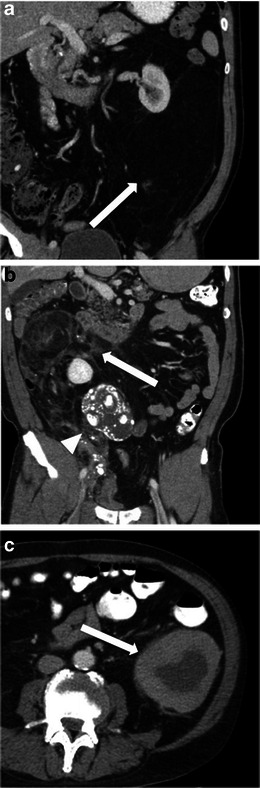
Imaging of liposarcoma a A 62-year-old woman with biopsy-proved well-differentiated liposarcoma. Note the large left retroperitoneal mass, which is of predominantly fatty attenuation (*arrow*). b A 56-year-old man with dedifferentiated liposarcoma. Coronal contrast-enhanced CT shows a large fatty right retroperitoneal mass with areas of soft tissue density (*arrow*) and coarse calcification (*arrowhead*). c Pleomorphic liposarcoma in a 60-year-old man. Note the enhancing retroperitoneal soft tissue mass with central necrosis (*arrow*)
